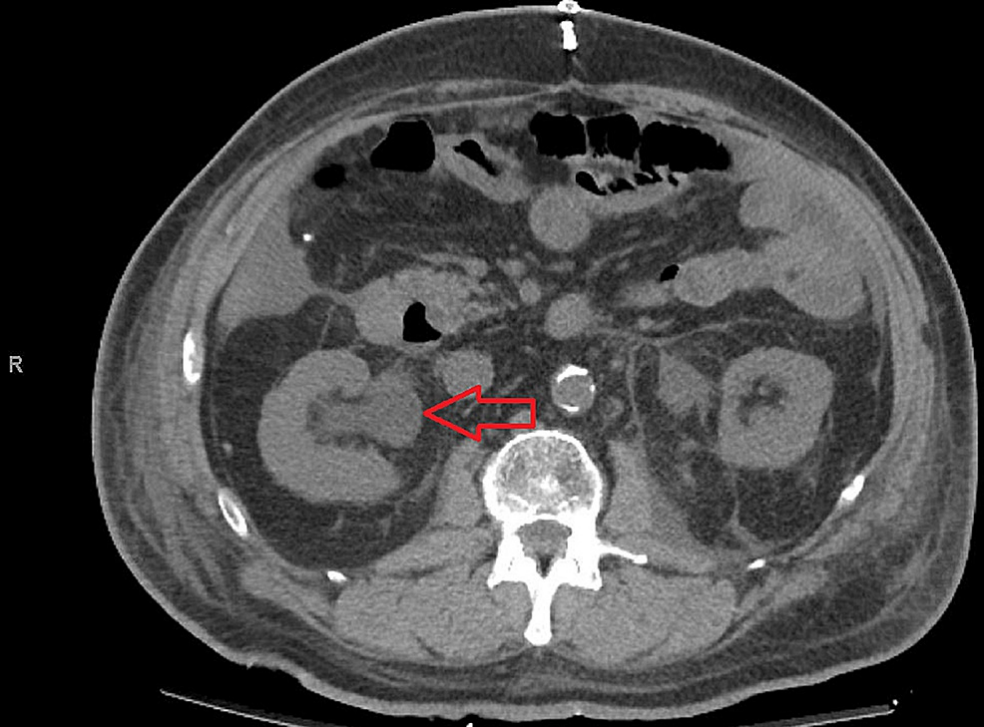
Noncontrast CT scan of the abdomen in our patient. Right renal pelvis showing an increase in size (red arrow).
